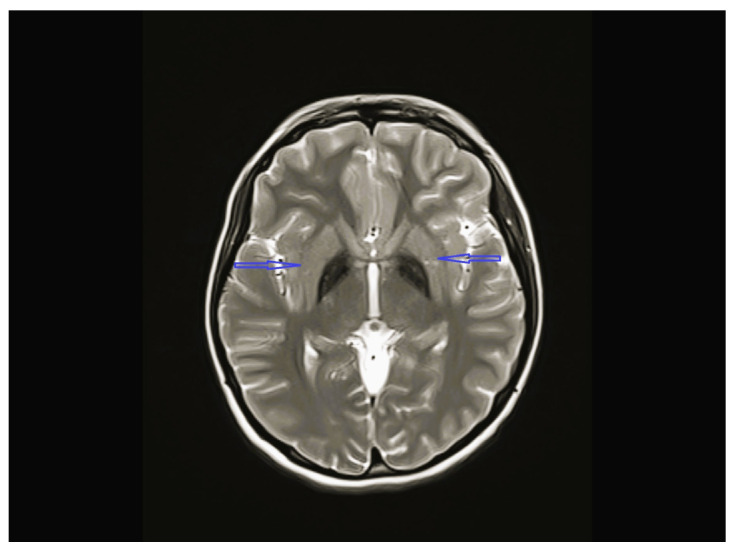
Brain MRI of case 4 revealing bilateral globus pallidus, T2 hypointensity and iron accumulation.
